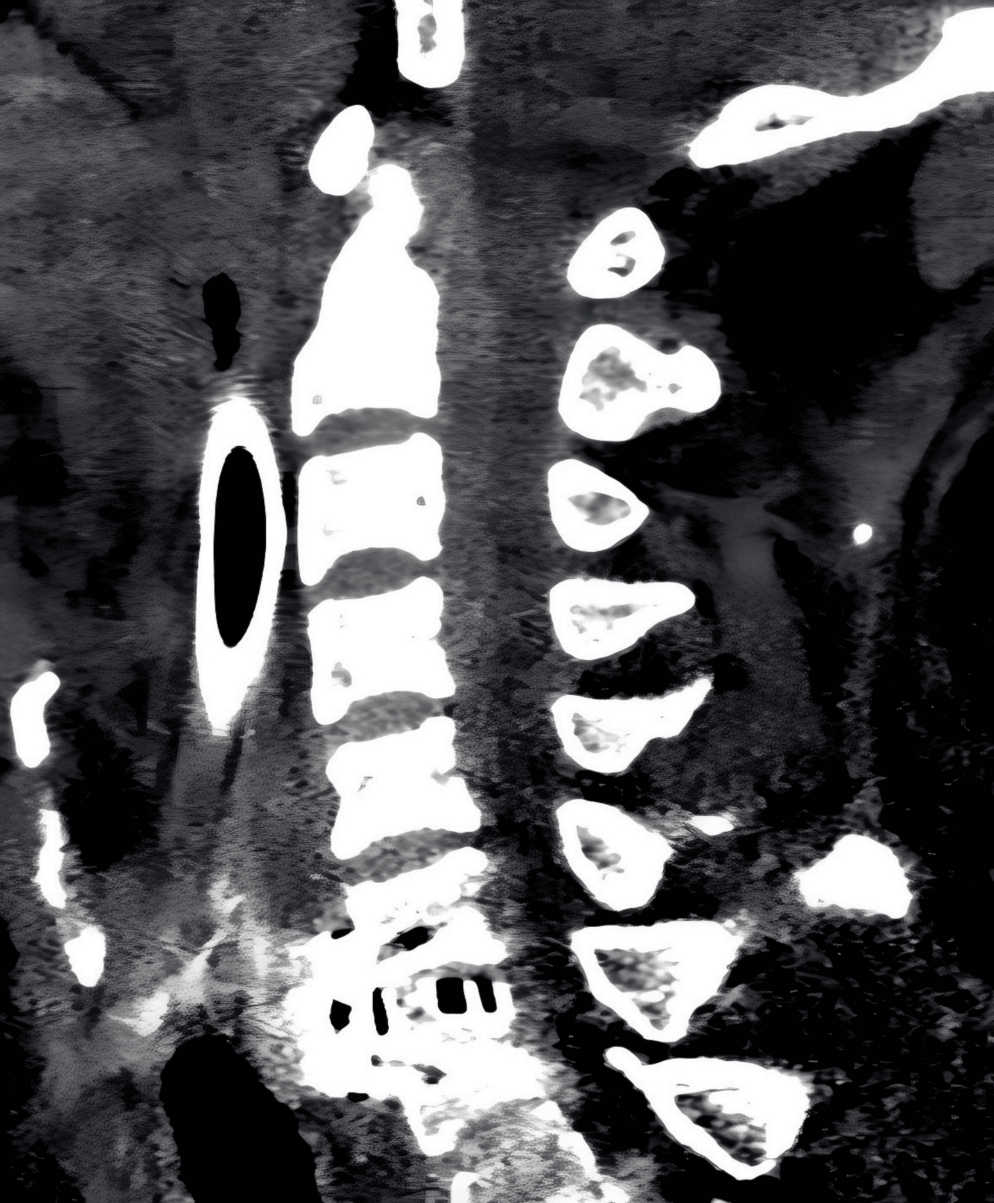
Cervical computed tomography (CT) scan performed emergently after the onset of spinal epidural hematoma (SEH) It reveals an epidural hematoma extending from C1 to C7, with significant compression of the cervicomedullary junction.
